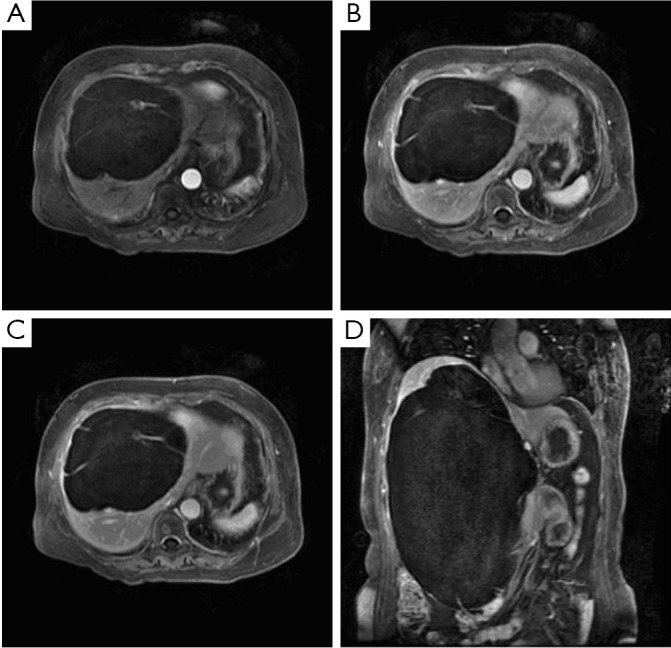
MRI-scan revealed a large cystic lesion in the liver. A. Arterial phage; B. Portal venous phage; C. Equilibrium phage; D. Coronal plane scan. A lesion of 27.9 cm × 17.9 cm × 14 cm in size occupied almost the entire liver with a thick-walled septus and cystic structure. The slight enhancement of the septum was observed after the injection of the intravenous contrast agent.
